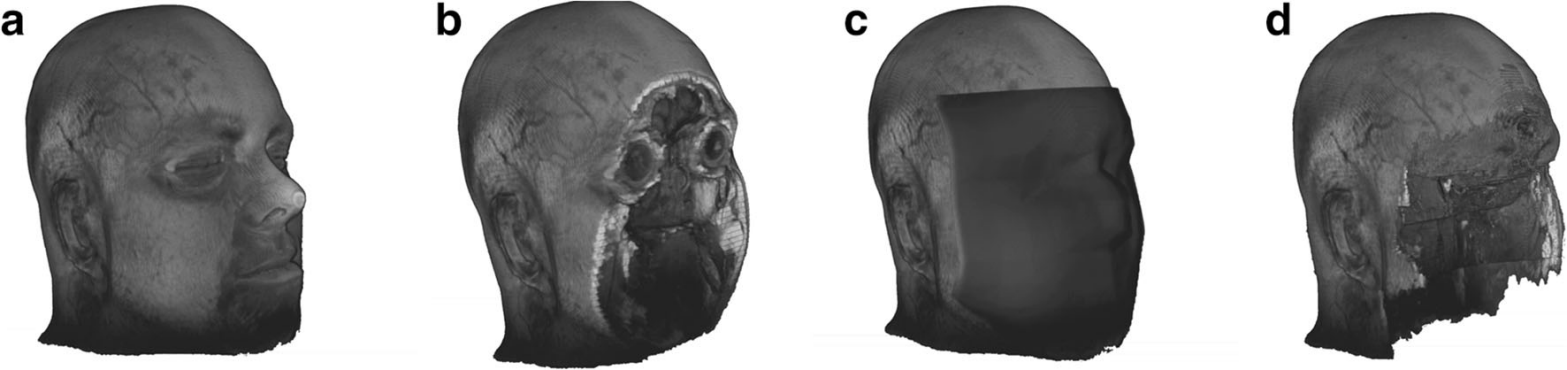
Example 3D-rendered MRI: full (a) and after removal of facial features with QuickShear (b), FaceMasking (c), and Defacing (d). The subject gave written informed consent for using data and for displaying rendering in this figure
